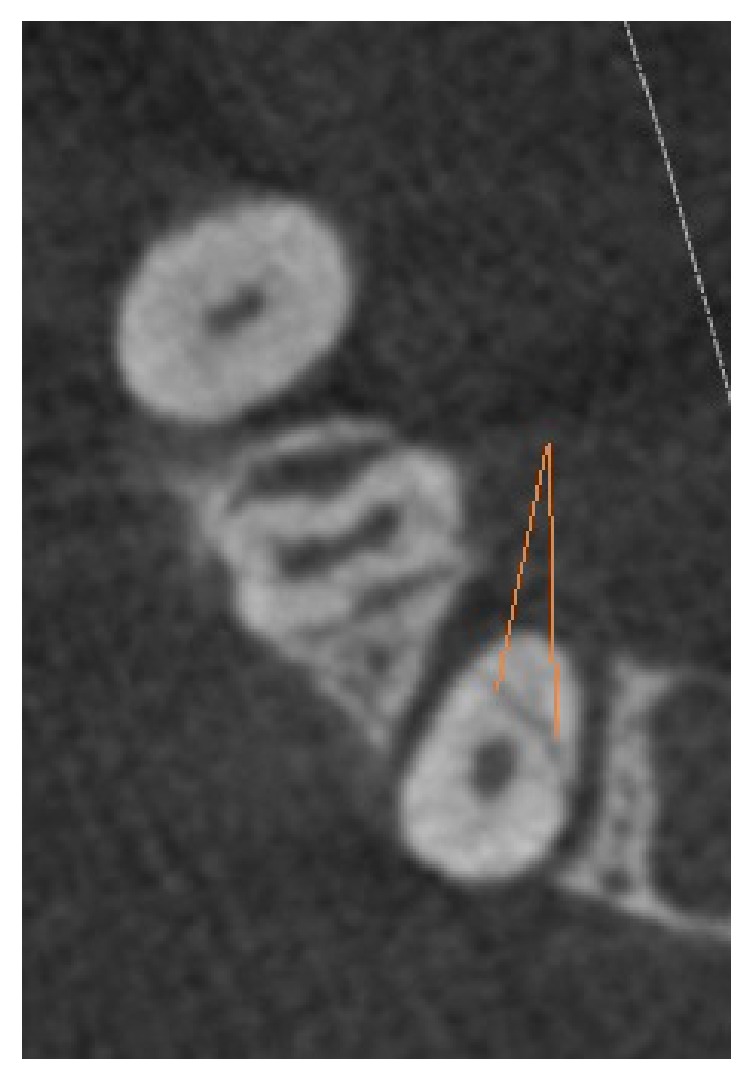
CBCT, axial view; red lines: VRF in empty canals (without gutta-percha).
